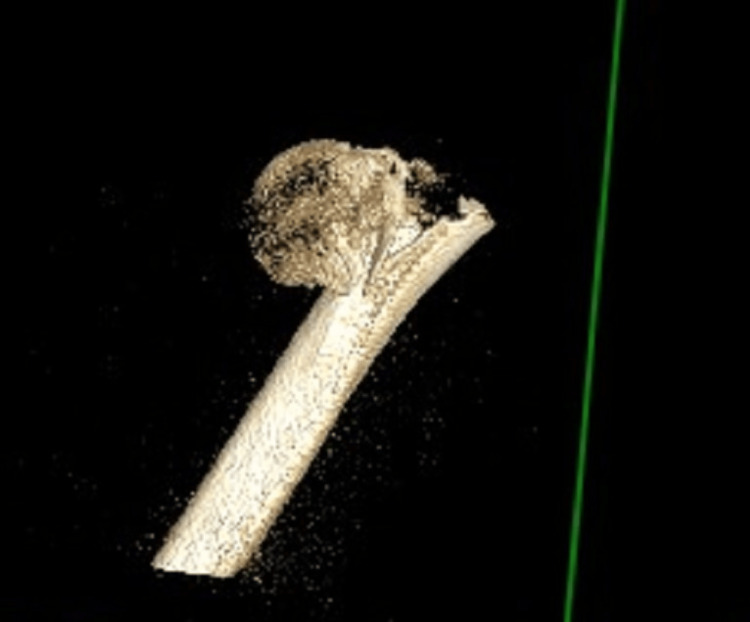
CT reconstruction of the proximal humerus fracture This demonstrates a highly comminuted and varus-displaced proximal humeral fracture. The lateral shaft fragment had lacerated the deltoid and had button-holed through it, as was noted in the operating theatre.
